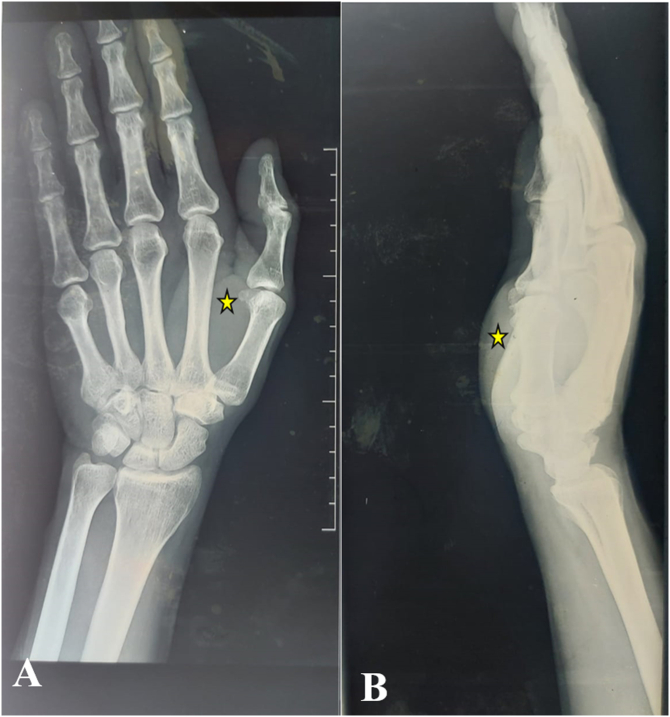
(A + B): A standard left hand radiograph revealed increased soft tissue density (), with no evidence of calcifications or associated bone lesions.
